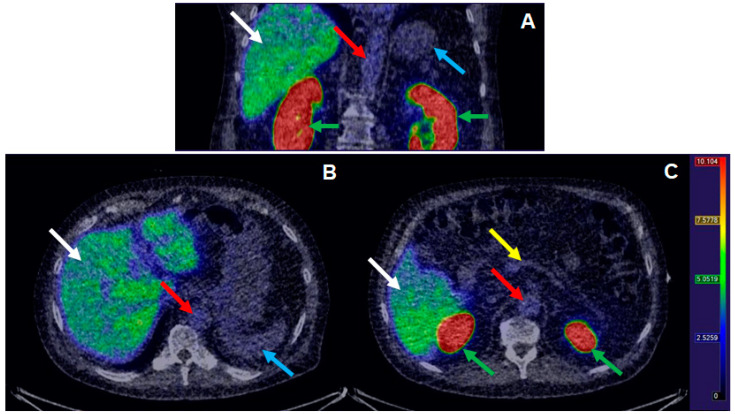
Biodistribution of [^68^Ga]Ga-DO3A-Tuna-2 in selected tissues after IV administration in patient (39 MBq, 0.45 MBq/kg, 10.2 µg peptide, 0.12 µg/kg peptide). Representative coronal (A) and transaxial (B,C), images of the abdomen. Images are summations of 20–60 min after administration and normalized to SUV 10. White arrows indicate liver, red aorta, blue spleen, yellow pancreas, and green kidney.
